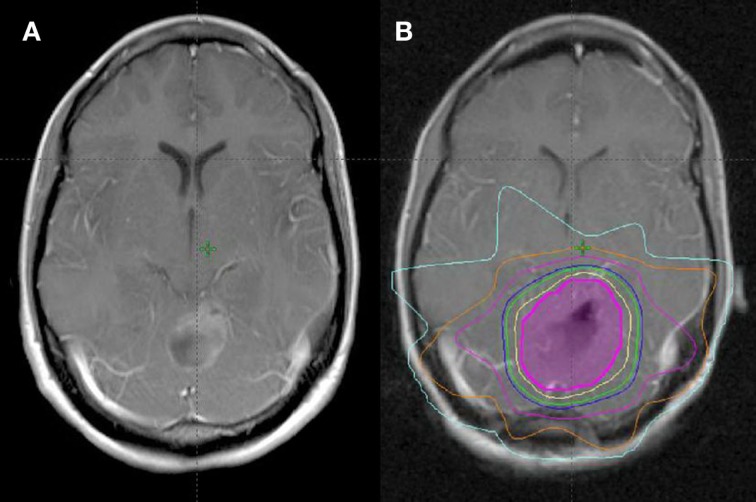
Treatment planning following GTR of single BM. (A) Pre-operative MRI (T1 post). (B) Post-operative MRI fused with planning CT for treatment planning (magenta = CTV, yellow = 100% isodose line, green = 95% isodose line, blue = 90% isodose line).
